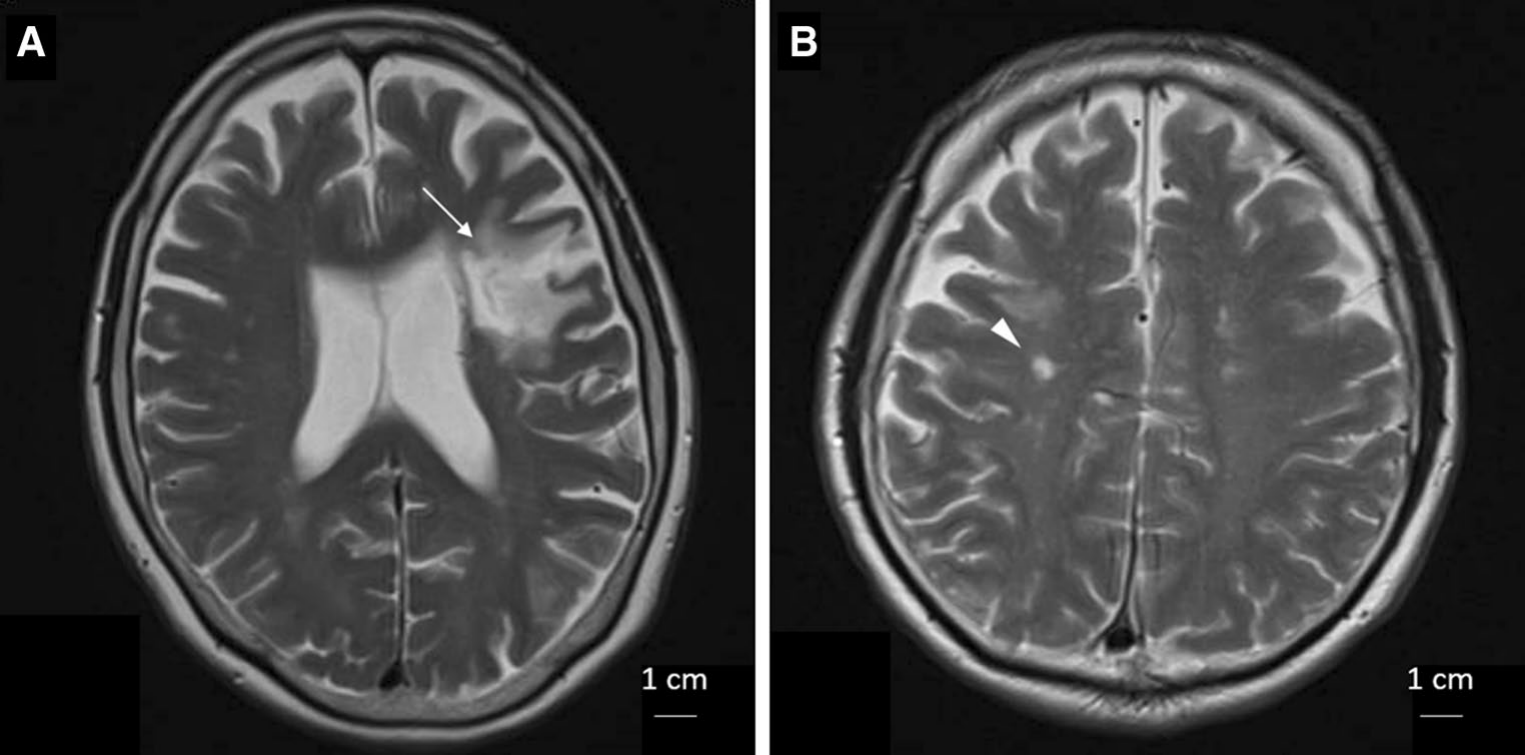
Representative MR images of the statin and no-statin patients. The infarct volume was measured as a 13.8 cm^3^ in a 60-year-old female without previous statin (arrow) and b 1.9 cm^3^ in a 69-year-old male with previous statin (arrowhead)
